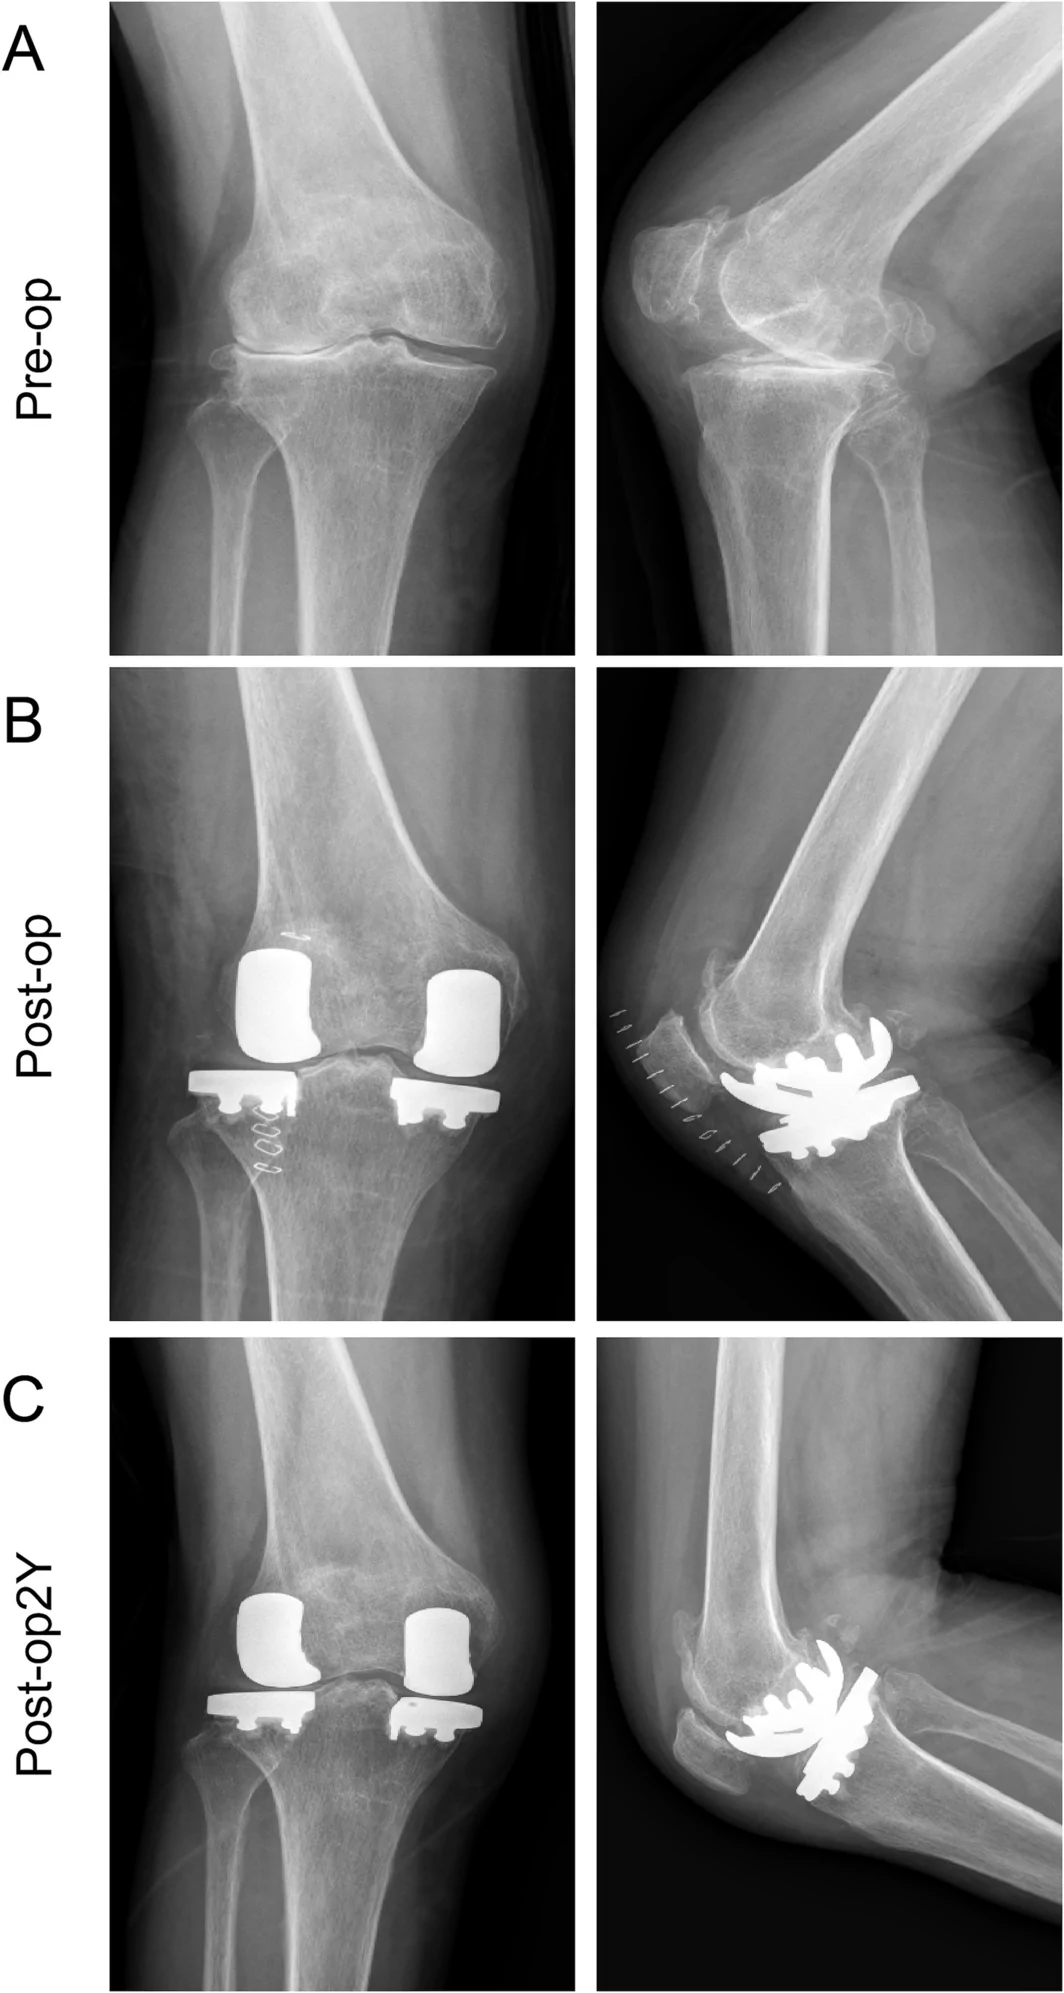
Representative radiographic follow‐up of another patient undergoing bicompartmental unicompartmental knee arthroplasty (bi‐UKA). (a) Preoperative anteroposterior (AP) and lateral radiographs demonstrate marked joint‐space narrowing in both the medial and lateral tibiofemoral compartments, accompanied by osteophyte formation and irregularity of the articular surfaces, consistent with bicompartmental knee osteoarthritis. (b) Immediate postoperative AP and lateral radiographs show satisfactory positioning and alignment of the medial and lateral unicompartmental components, with appropriate component congruency. (c) AP and lateral radiographs obtained 2 years postoperatively demonstrate maintained component position and alignment, without obvious radiographic evidence of prosthetic loosening, migration, periprosthetic fracture, or osteolysis, indicating a favorable mid‐term radiographic outcome.
